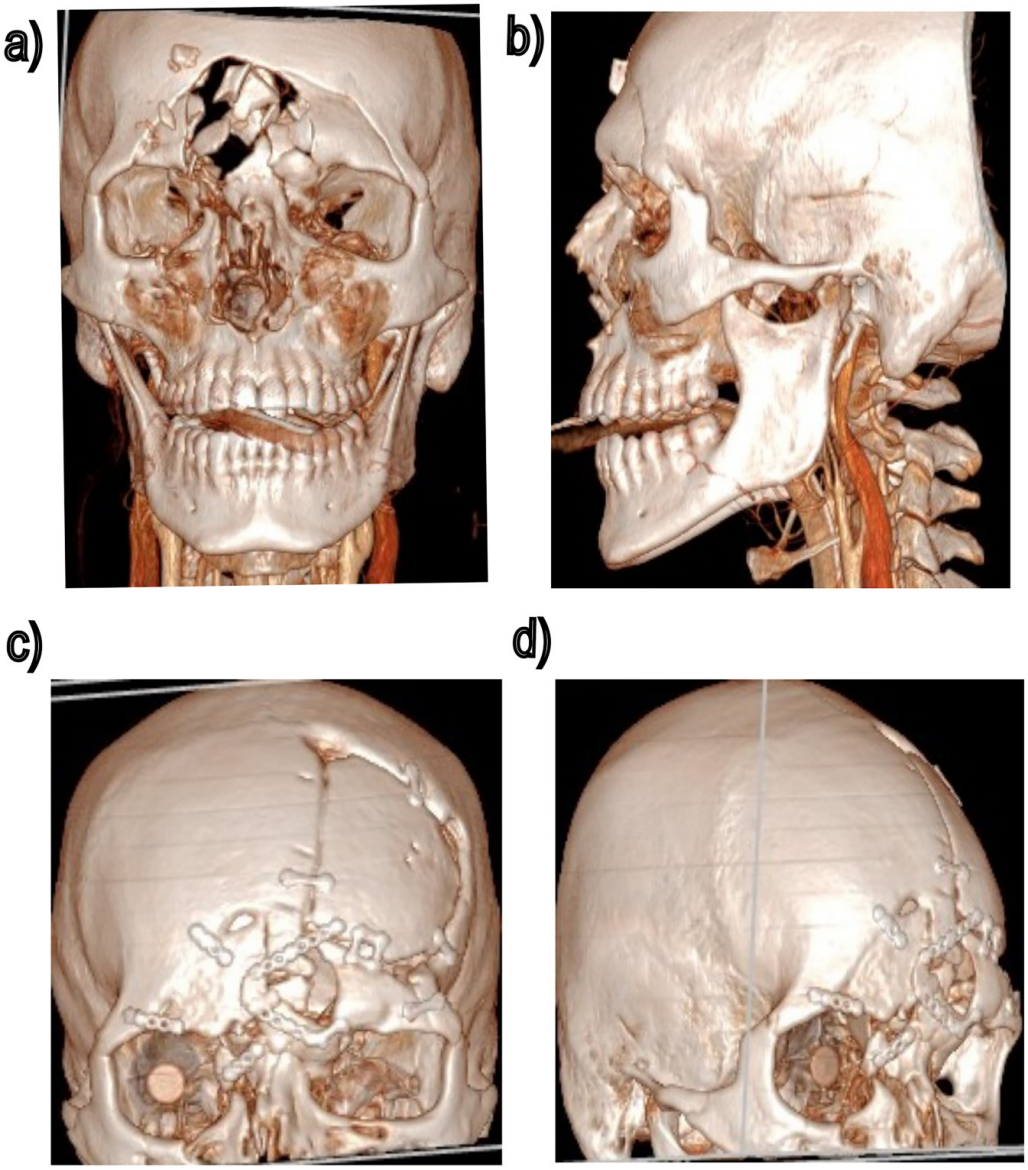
(a, b) Three-dimensional computed tomography (3D CT) scans of the primary injury. (c, d) 3D CT scans after calvarial reconstruction.
